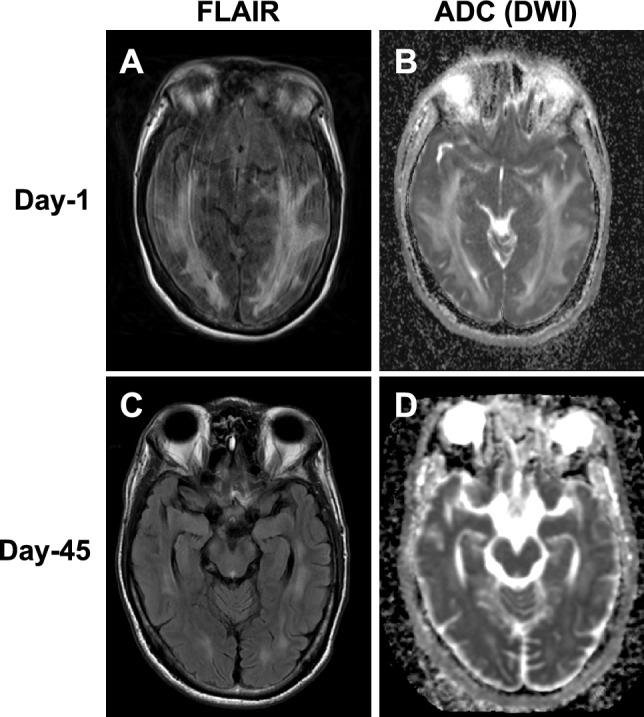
Brain magnetic resonance imaging scan. A The fluid attenuated inversion recovery (FLAIR) images showed high-intensity zones in the bilateral occipital lobes on the 1st hospital day. B Diffusion-weighted imaging (DWI) scans revealed increased apparent diffusion coefficient (ADC) These abnormalities were resolved on the 45th hospital day (C and D)
